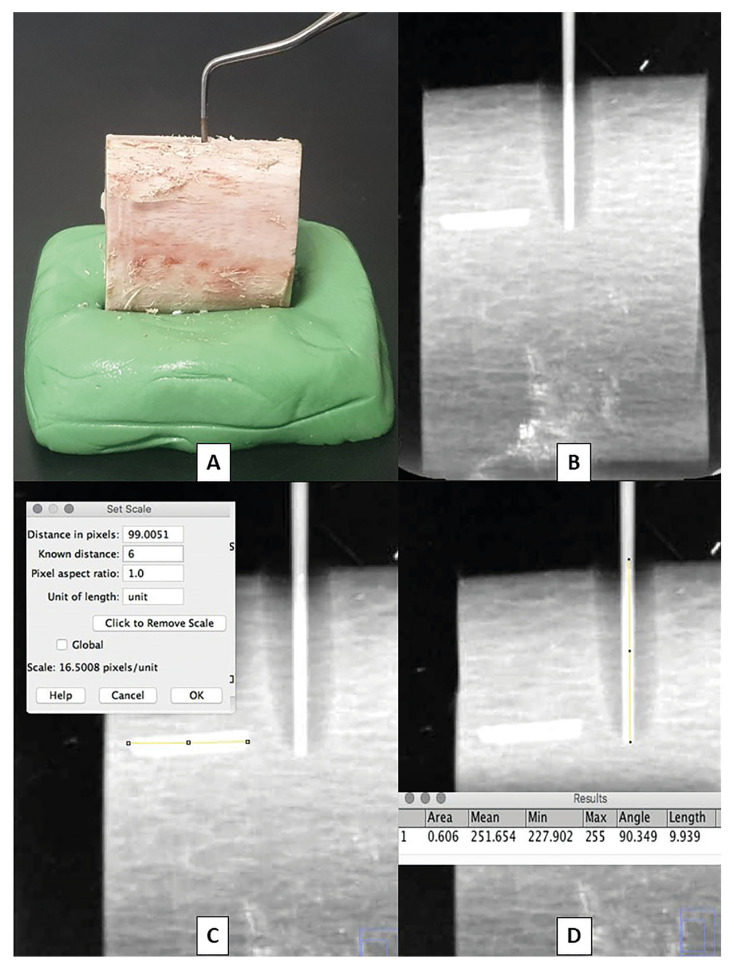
Clinical and radiological quantification of osteotomy depth. A: Clinical measurement of the osteotomy depth using a periodontal probe. B: Digital radiograph of the bone block after inserting a gutta-percha cone into the osteotomy and placing a 6 mm long metal segment on the anterior side of the block to calibrate the radiological images. C: Measurement with ImageJ® of the know distance of the metal segment. D: Measurement with ImageJ® of the depth of the osteotomy.
